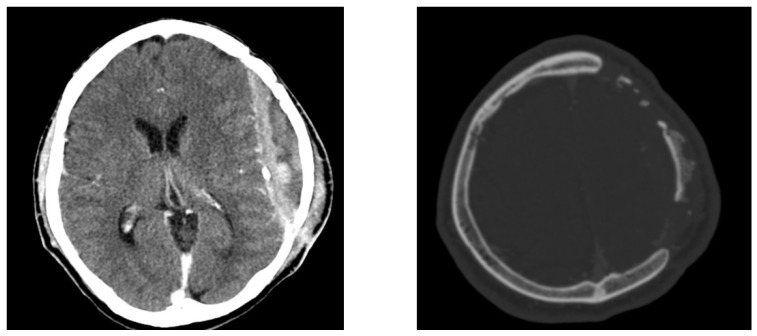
Brain contrast-enhanced computed tomography images show erosion of the bone flap and rim enhancement of the fluid collection. Physical examination did not reveal any tenderness, swelling, or wound dehiscence at the time of admission to the ER. However, squeezing of the left temporal incision site of the previous cranioplasty surgery caused the release of a small amount of purulent discharge. On neurological examination, the patient had a clear mental status and no neurological deficits; however, he was aphasic and murmured only simple words. On admission to the ER, his body temperature was normal (36.6 °C). Laboratory examination of infection markers revealed normal erythrocyte sedimentation rate (ESR), C-reactive protein (CRP) level, and white blood cell (WBC) count. The purulent discharge culture was negative for bacteria. Brain CT demonstrated an organized epidural abscess and resorption of the autologous implanted bone (Figure 3).
